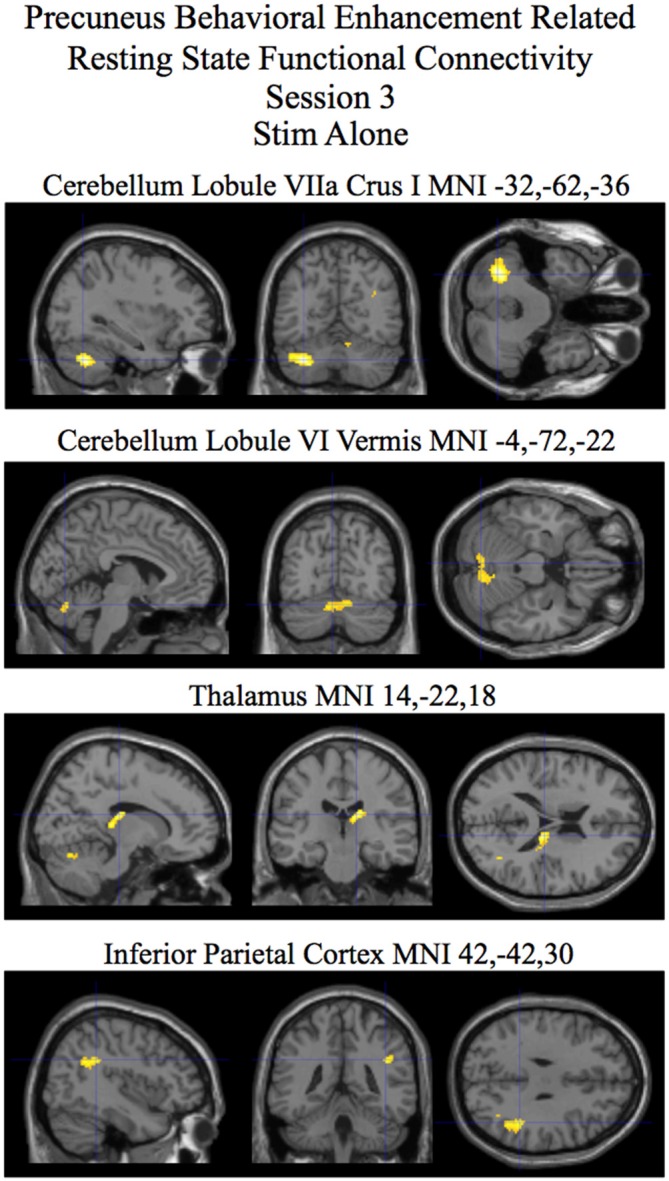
Session 2 results of the SPM random effects *t*-test for the stim alone contrast for resting state functional connectivity with the precuneus using post-pre behavioral performance as a covariate of interest. Statistically significant (*p* < 0.05 corrected) behaviorally related resting state functional connectivity is rendered on sections of a template T1 MRI scan at MNI coordinates for the peaks in the various significant clusters. Negative “*x*” MNI coordinates denote left hemisphere and positive “*x*” values denote right hemisphere activity. For the MRI sections the right side of the image is the right side of the brain.
